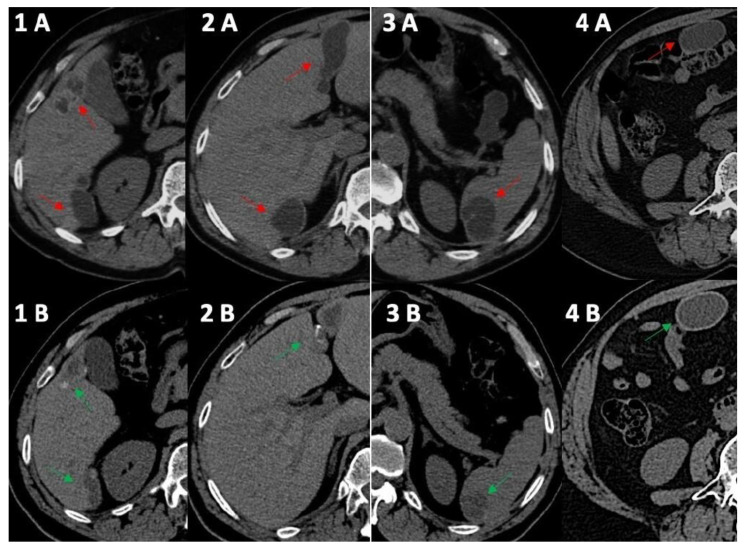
Computed tomography comparative imaging of some Echinococcus cysts in 2011 (A, red arrow) and in 2021 (B, green arrow). The cysts are located in liver segments 5 ((1A) 15 × 35 mm vs. (1B) 15 × 20 mm) and 6 (1–(2A) 41 × 45 mm vs. (1B) 10 × 36 mm), in segments 3–4 ((2A) 20 × 68 mm vs. 2B 19 × 45 mm), in the upper pole of the spleen ((3A) 30 × 40 mm vs. (3B) 30 × 30 mm), and in the right inframesocolic space ((4A) 41 × 22 mm vs. 4B 40 × 26 mm). In 2021, CT scans (1B,2B,3B) showed some initial signs of cystic wall calcifications that were not present in 2011 (1A, 2A, 3A). Uncertainty persisted for the right inframesocolic cyst (4B), but in 2023 (Figure 2, #3), US demonstrated signs of intracystic degenerative content (Figure 2—n3).
